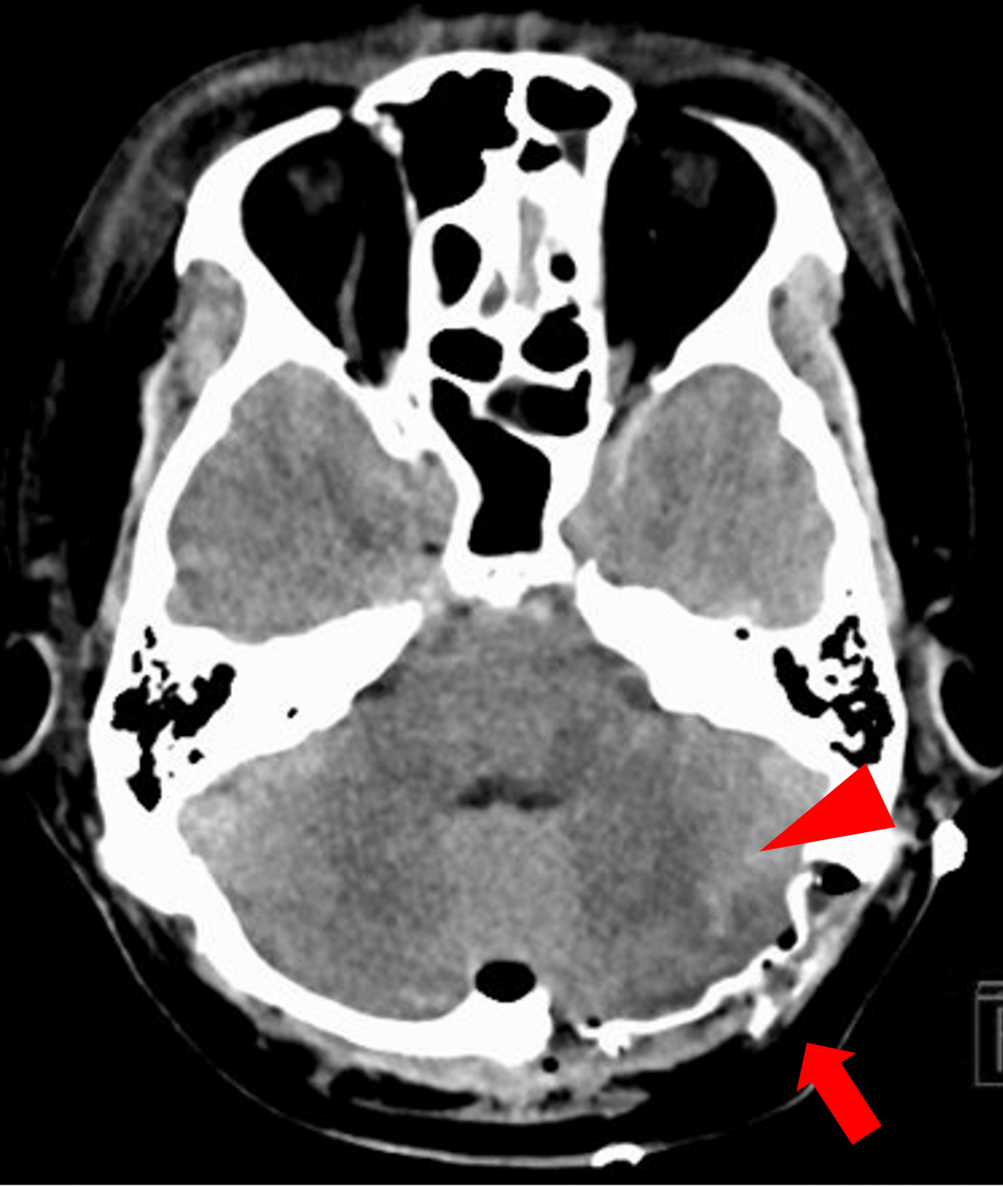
Postoperative CT after aneurysm trapping and posterior fossa decompression. Postoperative non-contrast head CT showing decompressive craniectomy without bone flap replacement (arrow). Suspected infarction in the left posterior inferior cerebellar artery (PICA) territory is noted (arrowhead).
